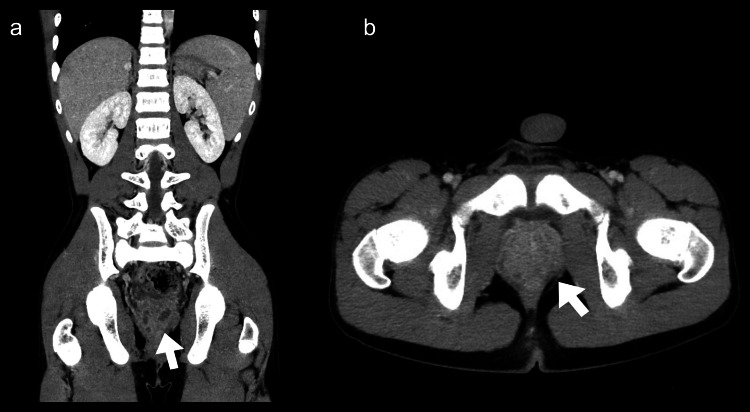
Contrast-enhanced computed tomography images of the patient (a) Coronal view, (b) axial view. The white arrows indicate diffuse enlargement of the prostate and numerous areas of poor contrast within the prostate.
